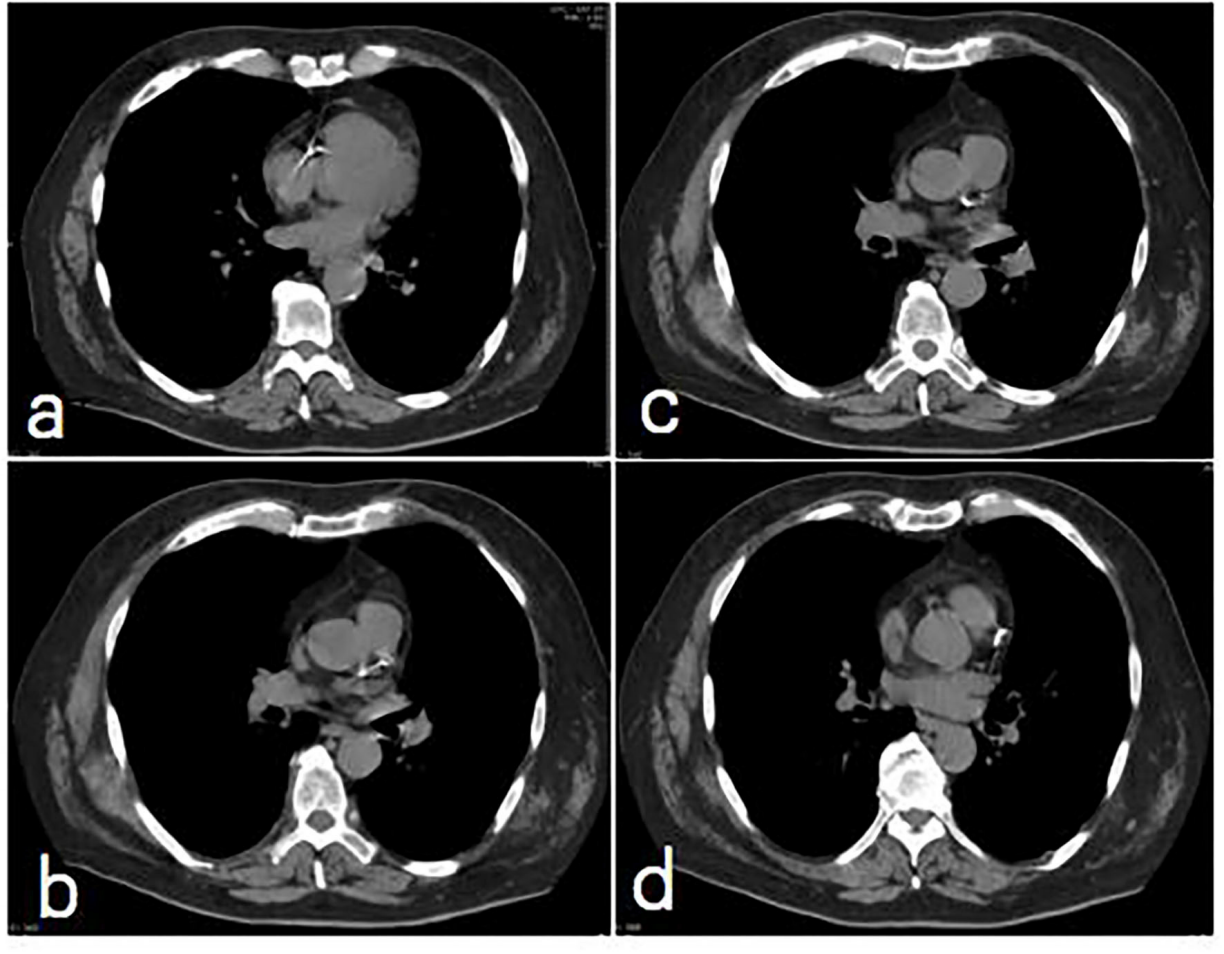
A non contrast, non-gated Chest CT image series for CAC (a) RCA calcification score = 3; (b) LM calcification score = 3; (c) mid-LAD score = 3; (d) proximal LAD calcification score = 3. The total CAC score = 9.
